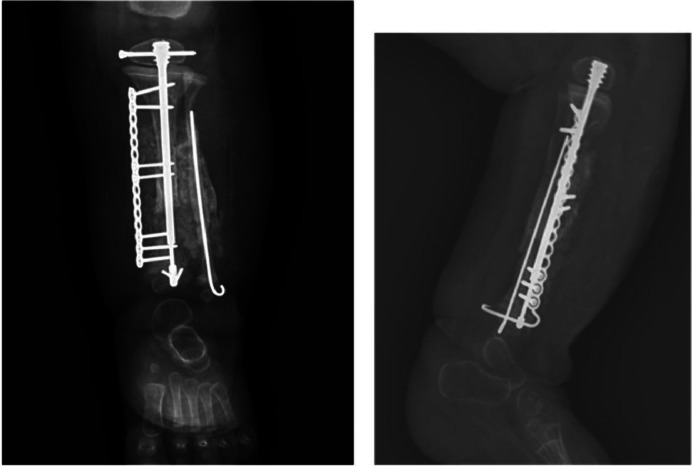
Radiograph of lower limb obtained after cross-union surgery shows good healing progress with properly positioned fixation implants.
